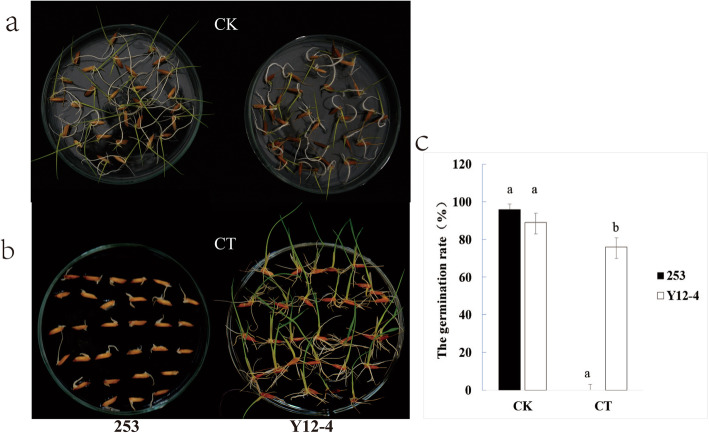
The comparison of the germination rate under low temperature of 253 and Y12–4 in the control and in the cold treatment. CK indicates control treatment; CT indicates cold treatment. Vertical bar indicates standard error. a: 253 and Y12–4 under 25 °C for 7d; b: 253 and Y12–4 under 4 °C for 10d and recovered for 5d; c: the germination rate of 253 and Y12–4 under normal and cold treatment
